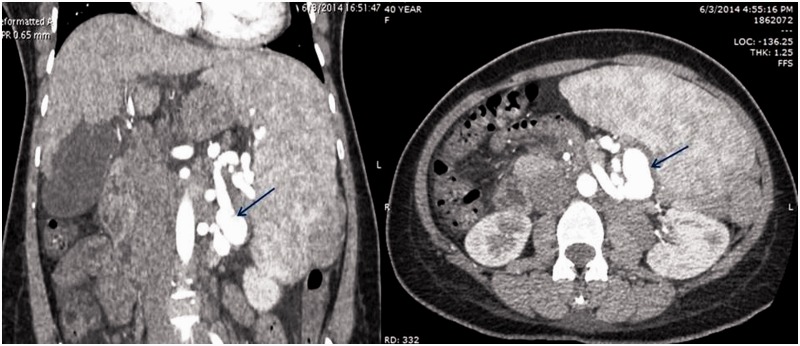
Computed tomography of the abdomen. Left to right: the presence of multiple splenic artery aneurysms in association with portosystemic collaterals (blue arrow).
